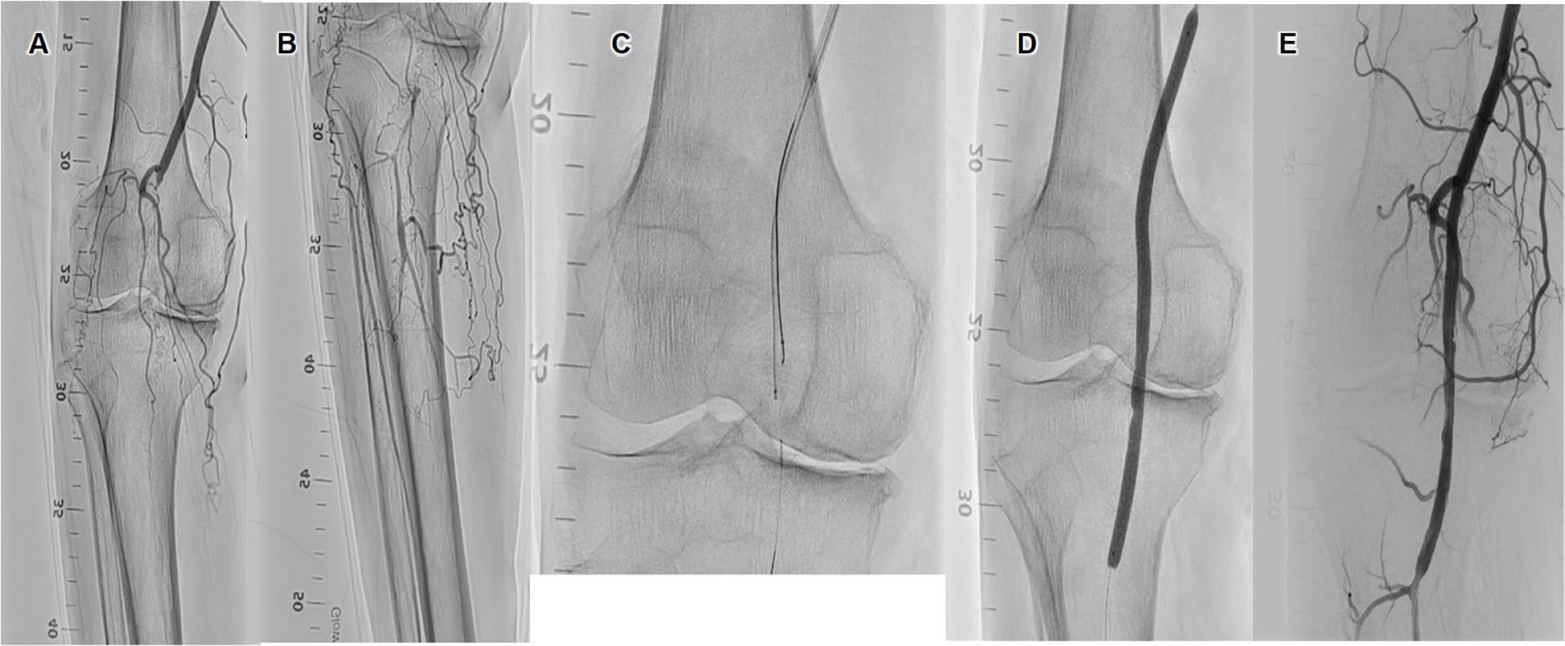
Angiogram of the endovascular treatment in Case 2. A Control angiography shows that the right popliteal artery is occluded from the proximal segment. B Vessels are contrasted from the distal portion through the collateral vessels. C Intravascular ultrasound-guided wiring is performed. D A 5.0- × 150-mm drug-coated balloon (Lutonix^®^; BD, USA) is dilated at the popliteal artery lesion to prevent restenosis. E The final angiography shows good antegrade flow with no dissection or residual stenosis
